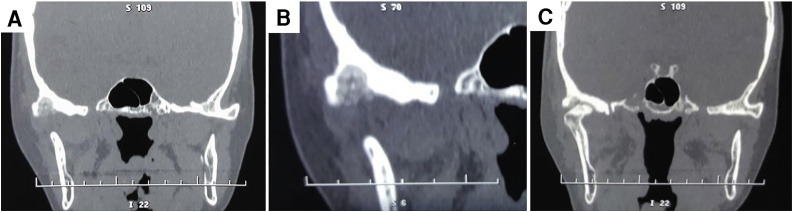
Coronal CT slice demonstrates a well-defined osteolytic lesion in the skull base (A). (B) Focused image shows a heterogeneous nidus contains multiple hyperdense masses with diffused sclerosis of the adjacent bone. (C) The lesion extends posteriorly into the glenoid fossa of the TMJ.
